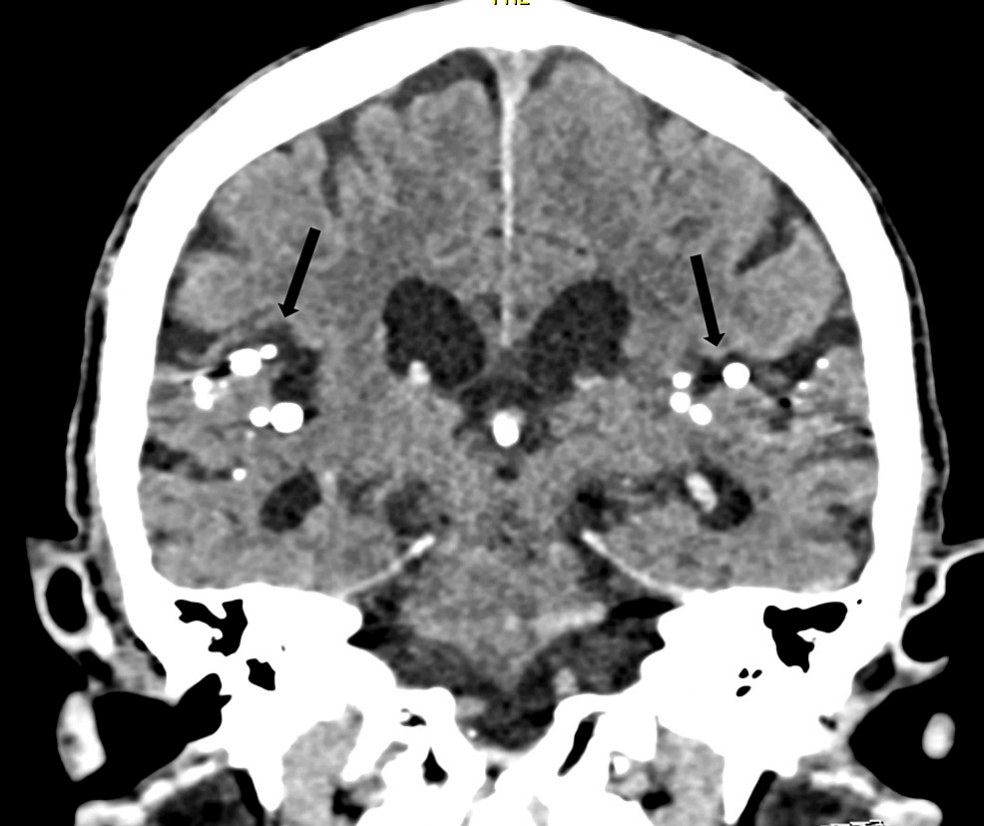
Coronal CT scan image of the skull. Coronal CT scan image of the skull showing multiple hyperdense droplets in the subarachnoid space of the Sylvian fissures (arrows).
